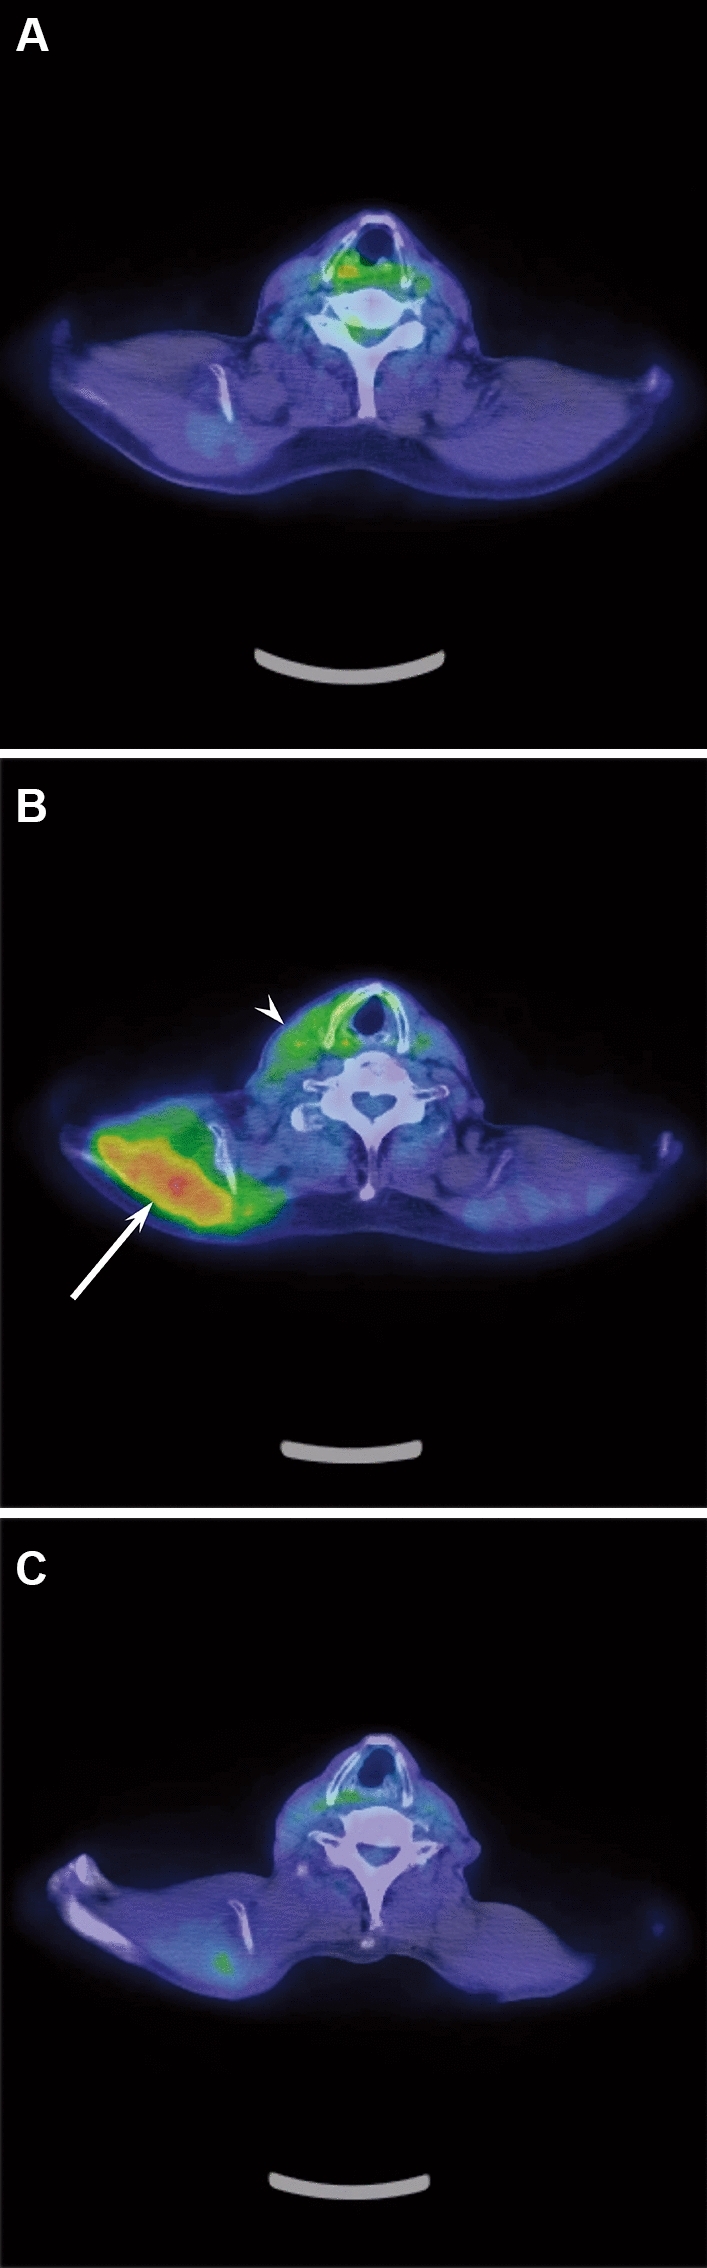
A 71-year-old man with right oropharyngeal cancer who underwent right unilateral modified radical neck dissection for levels I–V and right accessory nerve dissection. a Preoperative PET/CT image shows no accumulation of FDG in the cervical muscles. b Postoperative PET/CT image after 6 months of neck dissection shows FDG uptake in the right trapezius (SUVmax: 3.51) (arrow) and sternocleidomastoid (SUVmax: 2.25) (arrowhead) muscles on the dissected side. c Postoperative PET/CT image after 19 months of neck dissection shows decreased FDG uptake in the right trapezius and sternocleidomastoid muscles
